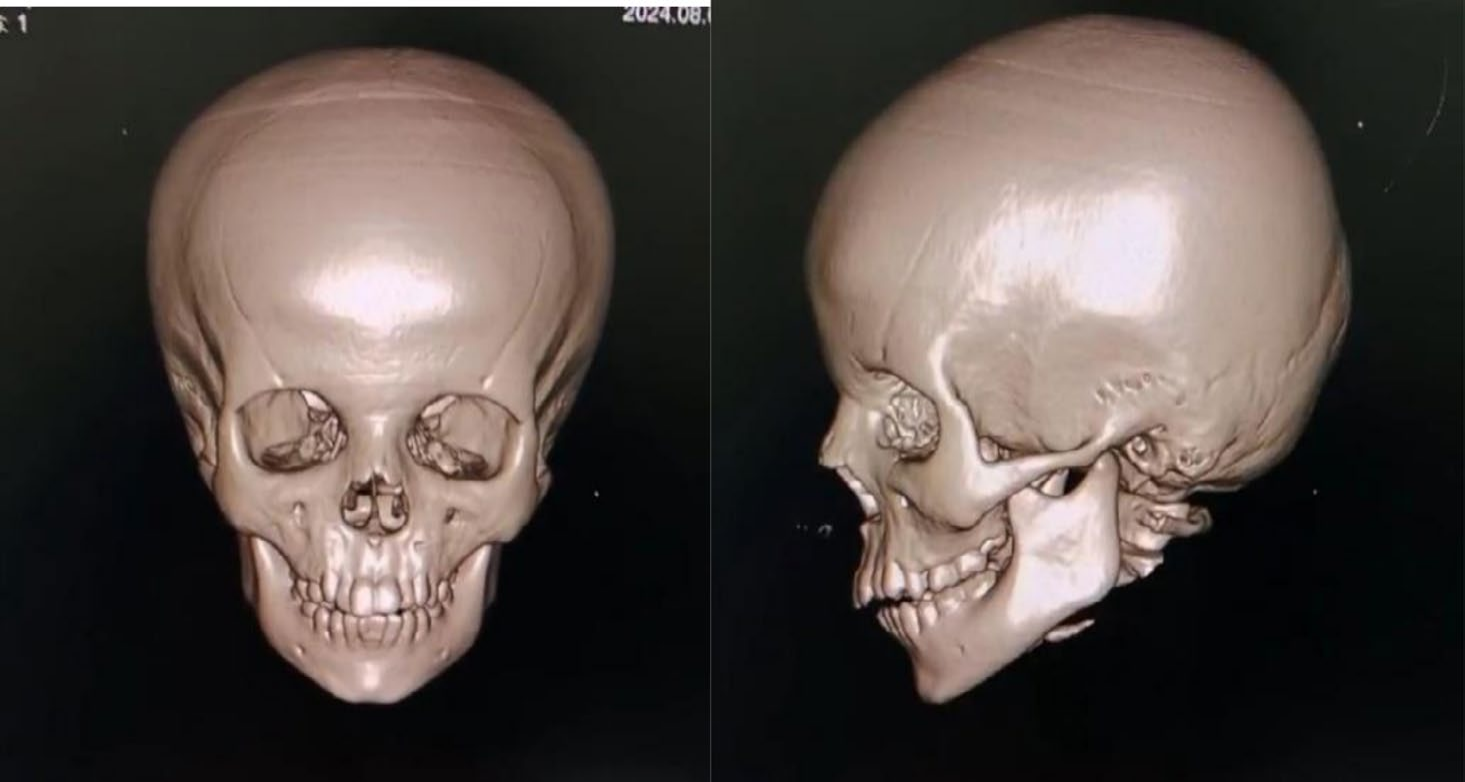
3D CT scan showing only soft tissue edema without any bone involvement.
